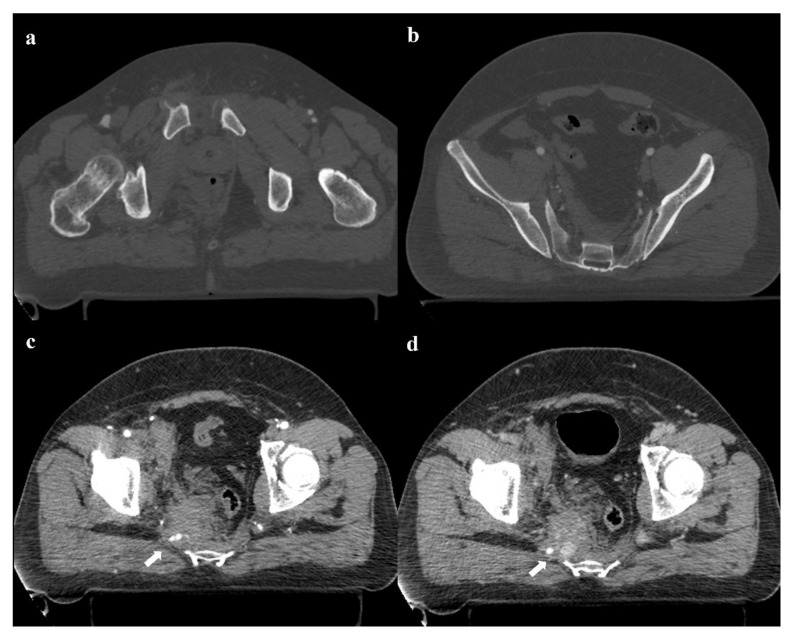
Axial CT images show a pubic symphysis diastasis of 2.9 cm, and an anterior widening of the right sacroiliac joint (a,b). Active extravasation of contrast medium in the arterial phase of the CT exam is seen near the right side of the fifth sacral vertebra (arrow in (c)). The extravasation increases in the next phase of the examination (arrow in (d)). This is acute arterial bleeding from a branch of the right inferior gluteal artery.
